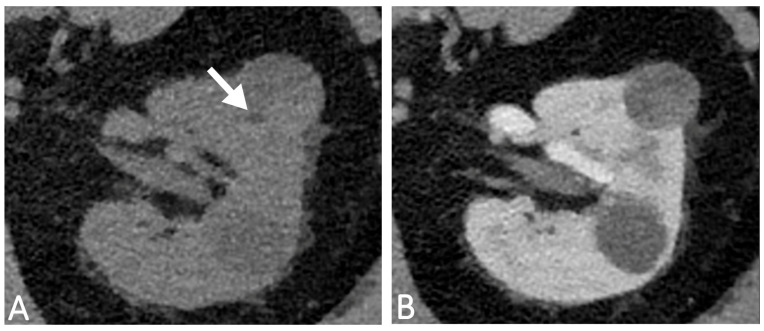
56-year-old man with a history of right nephrectomy due to multiple pRCC. Follow-up CT scans obtained before (A) and after contrast injection (B) show a 2.5 cm heterogeneous mass within the left kidney, with small hypodense focus not clearly related to fat (white arrow) and very subtle contrast enhancement. No calcifications within the mass were identified.
